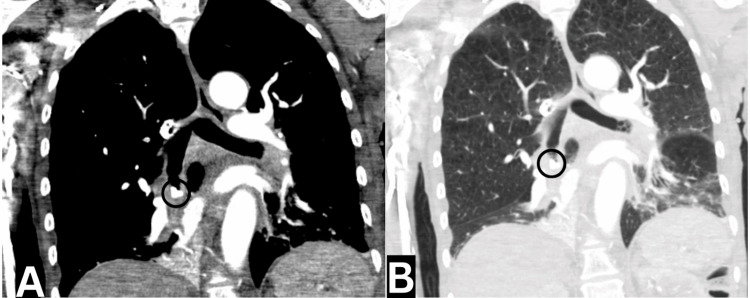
Computed tomography scan (coronal View) showing near-complete opacification of the right lower lobe: (A) mediastinum window and (B) lung window
